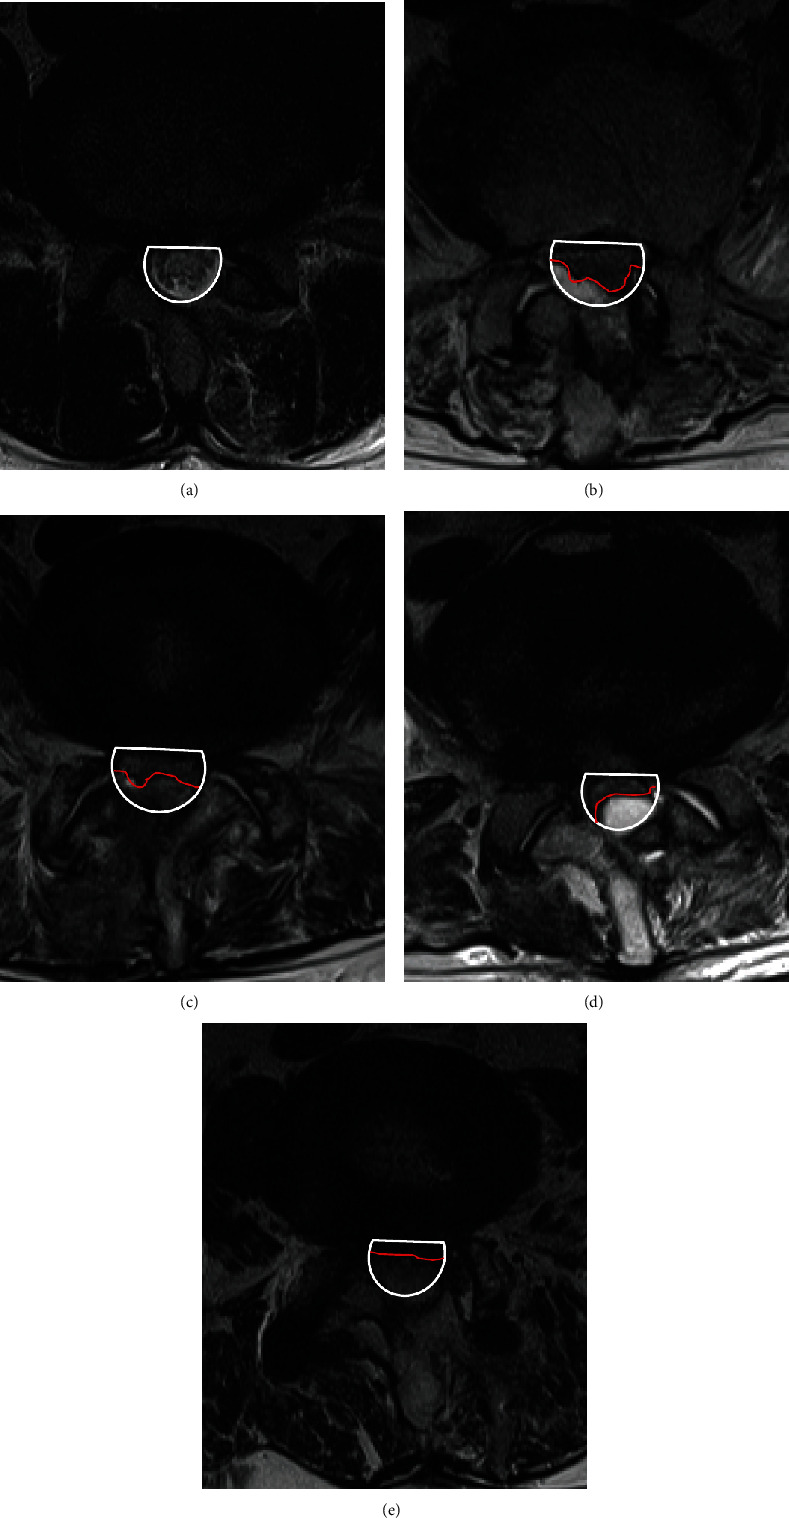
Measuring the severity of PSEH on T2-weighted axial MRI. (a) Grade 0, (b) Grade I, (c) Grade II, (d) Grade III, and (e) Grade IV. (PSEH: postoperative spinal epidural hematoma, MRI: magnetic resonance image).
